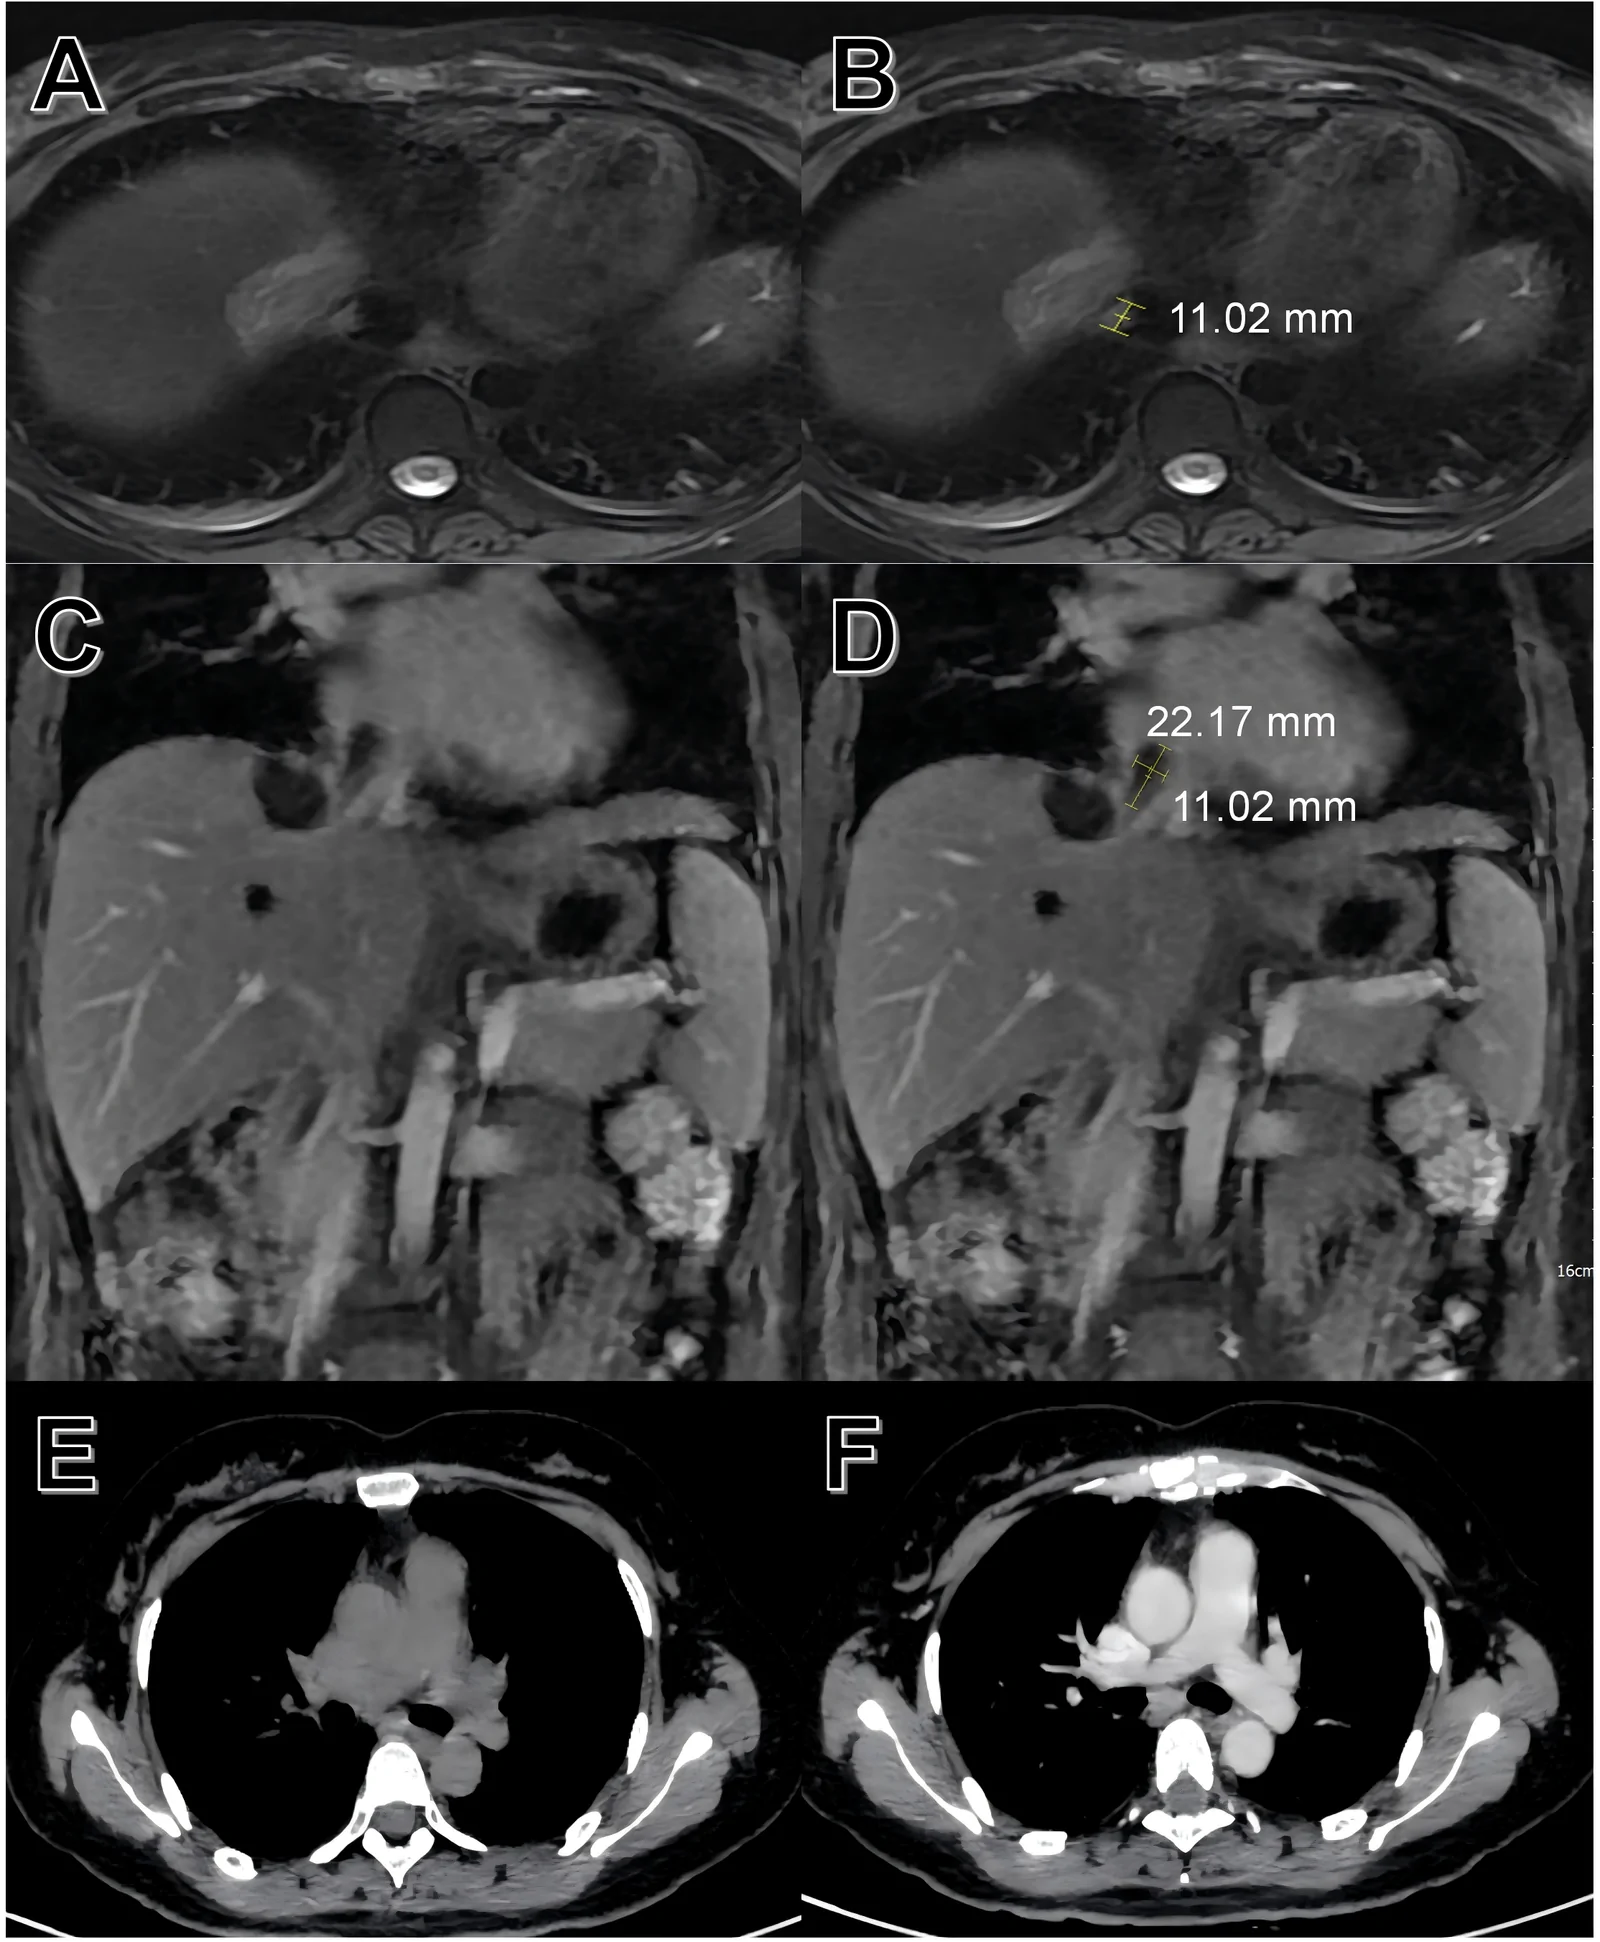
(A–D) abdominal magnetic resonance images obtained on November 27, 2025. (A, B) T1-weighted axial contrast-enhanced (T1-Ax-C+) images. (C, D) T1-weighted coronal contrast-enhanced (T1-Cor-C+) images. (E, F) CT pulmonary angiography images obtained on December 16, 2025.
